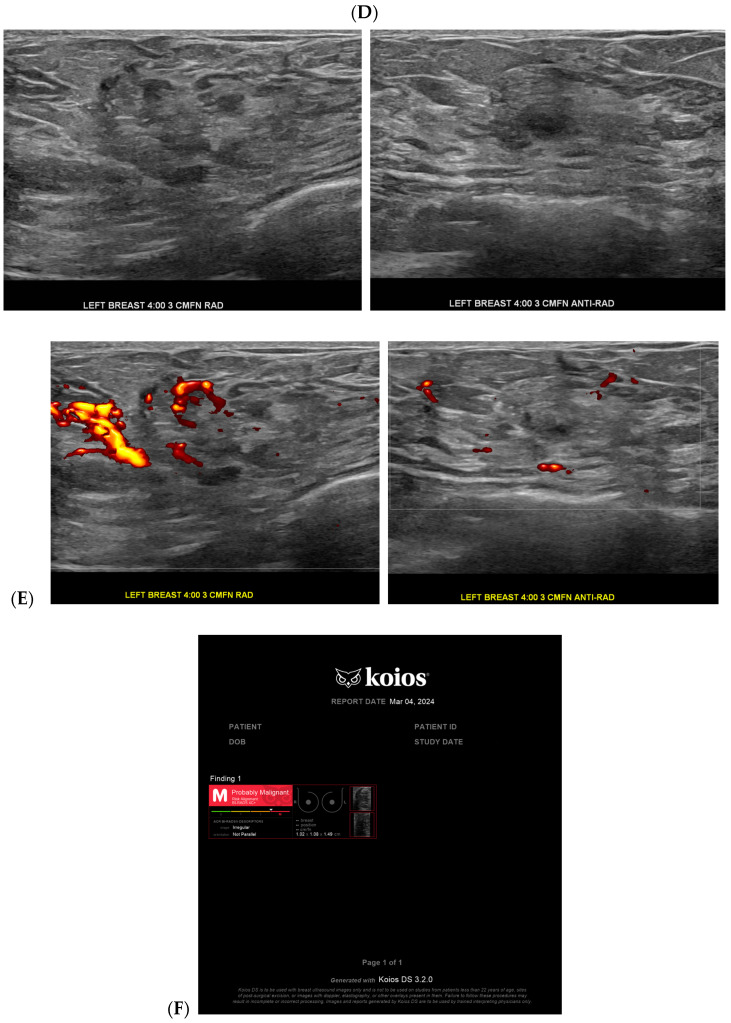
Developing asymmetry detected by artificial intelligence (AI): Between the baseline screening mammogram (A) and the follow-up screening mammogram 17 months later (B), there has been a very subtle development of left breast asymmetry that is difficult to perceive with the naked eye. However, the AI program Transpara highlighted potential regions of interest (C) for the radiologist to query for additional mammographic and sonographic imaging. On further diagnostic imaging, the subtle asymmetry corresponds to a hypoechoic mass at left 4:00, 3 cm FN (D) with hypervascularity (E). AI program Koios correctly recognized the mass as “Probably Malignant”, and this area returned as a biopsy-proven invasive malignancy with lymphangitic spread (F). Images obtained from the Icahn School of Medicine at Mount Sinai.
